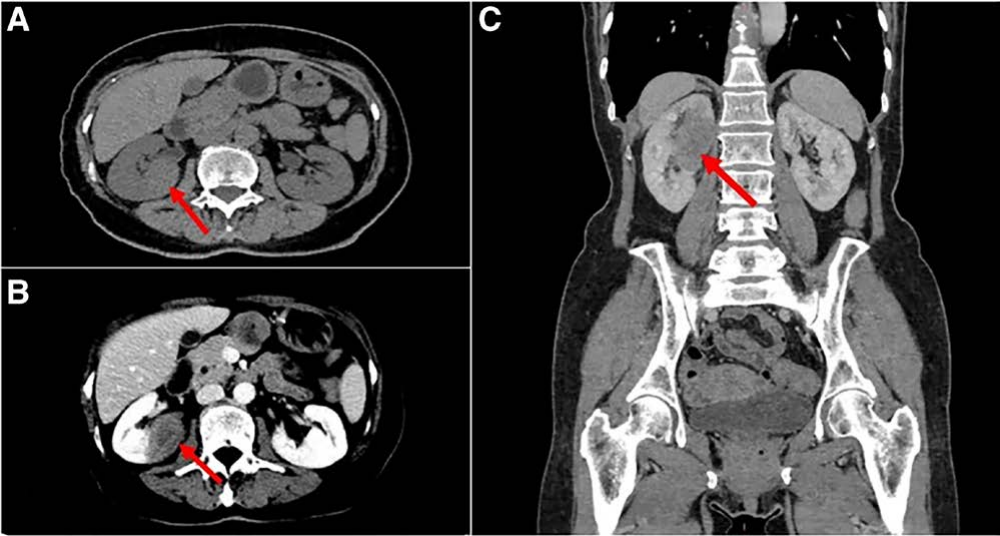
Computerized Tomography (CT) (slice thickness 0.9 mm). (A) Nonenhanced CT shows a homogeneous isodensity mass in the upper portion of right kidney (arrow). (B) Enhanced CT demonstrated mild enhancement in the cortical phase(arrow). (C) In the delayed phase, the enhancement of the mass increases(arrow).
